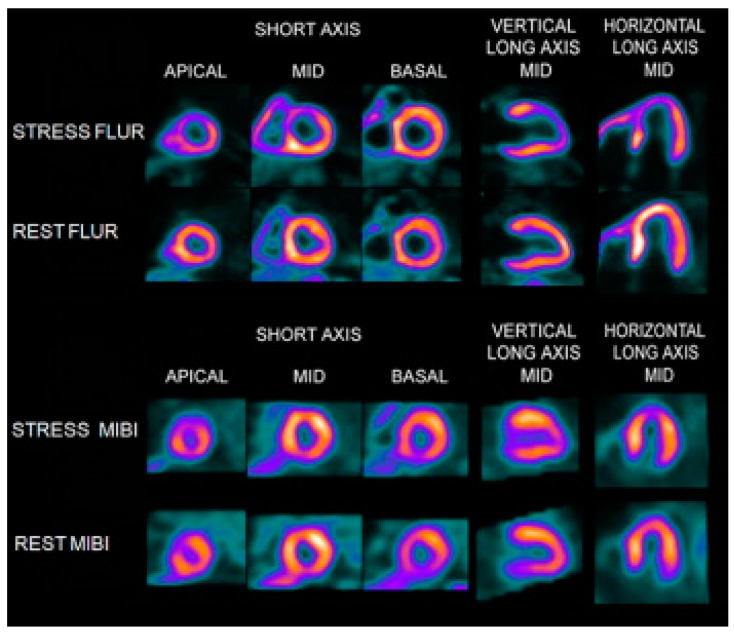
The ^18^F-flurpiridaz PET (top) and MIBI SPECT (bottom) images from an 82-year-old man with shortness of breath and an occluded native proximal left anterior descending (LAD) coronary artery and an occluded left internal mammary graft to the LAD and no other significant native CAD. The ^18^F-flurpiridaz images show a severe reversible perfusion defect throughout the territory of the occluded proximal LAD, whereas the MIBI images show only a moderate perfusion defect in the distal LAD territory (apical slices) [58].
